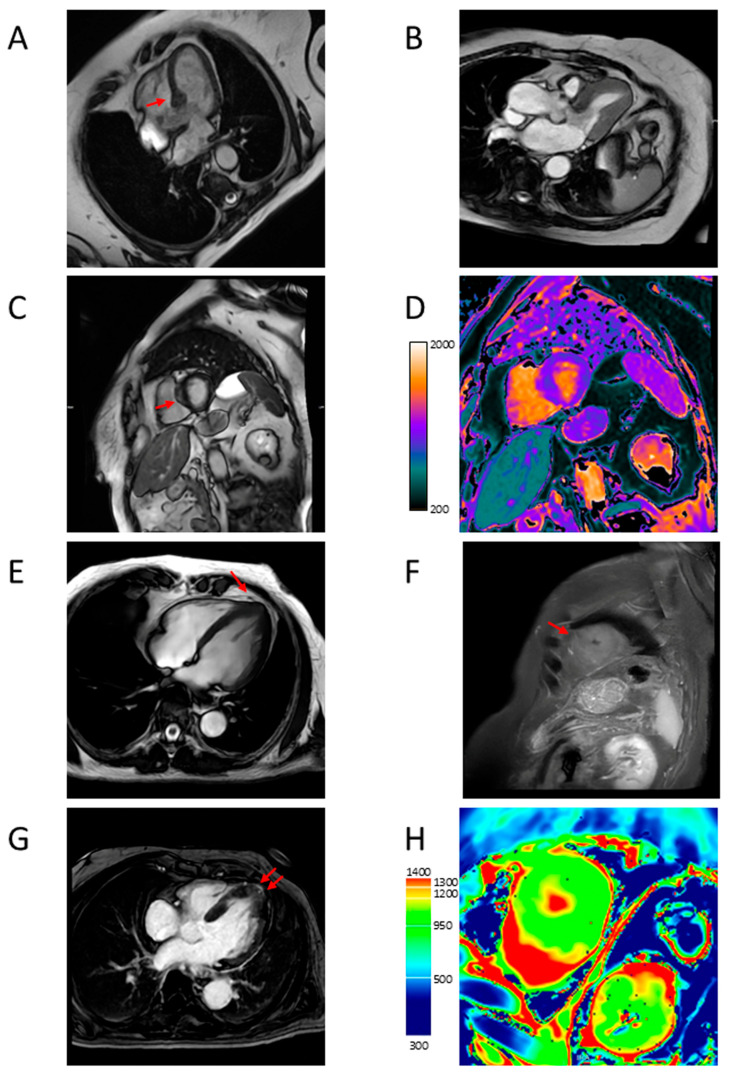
Representative CMR images from a female patient aged 74 years with mass-like hypertrophy (A–D) and a male patient aged 80 years with apical hypertrophy (E–H). (A) four-chamber long axis view showing mass-like asymmetric basal septal hypertrophy (arrow); (B) three-chamber long axis view showing LVOTO; (C) short axis view showing LGE with patchy distribution at the level of the hypertrophic segment (arrow); (D) short axis view showing increased T1 mapping values at the same level; (E) four-chamber long axis view showing asymmetric apical hypertrophy (arrow); (F) short axis T2-weighted view showing apical hyperintensity (arrow); (G) four-chamber long axis view showing apical LGE (arrows); (H) short axis view showing increased T1 mapping values at the same level. CMR = cardiac magnetic resonance; LVOTO = left ventricular outflow tract obstruction; LGE = late gadolinium enhancement.
